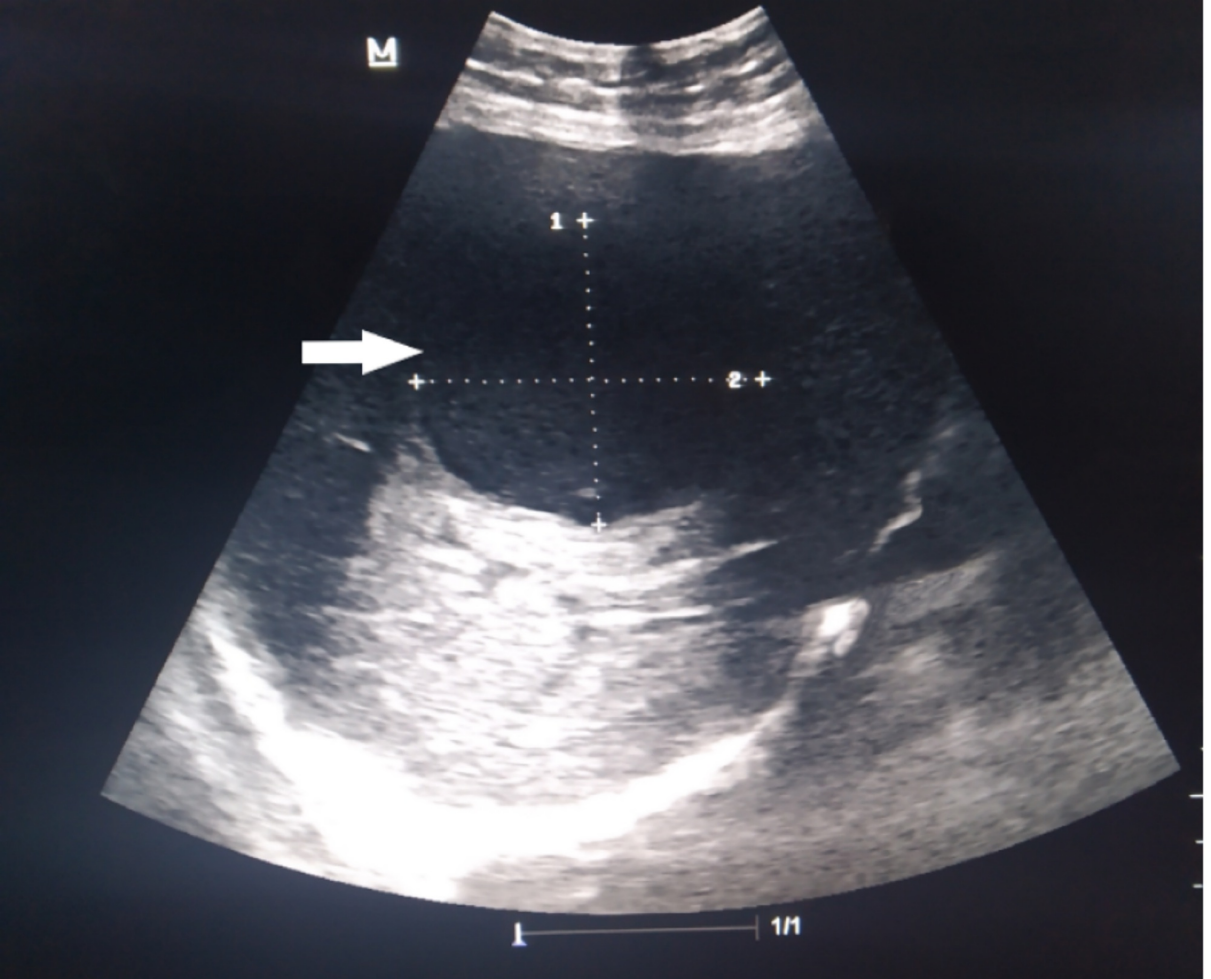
Ultrasound of the liver showing a hypodense lesion (arrow) in the right lobe.
